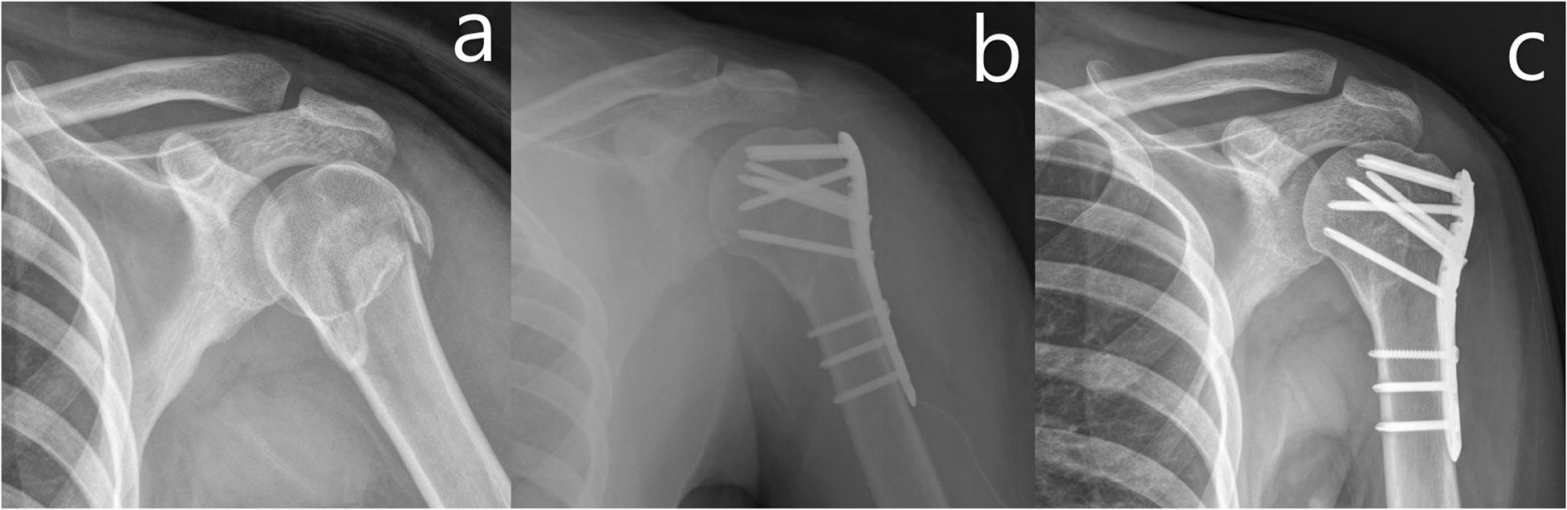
Simple radiographs of the conventional bicortical locking screw fixation. a Preoperative, b Postoperative and c 3 months after surgery
